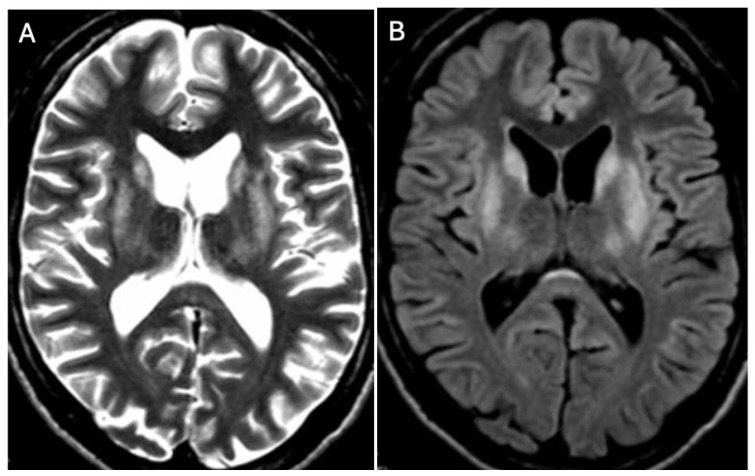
Hyperintense changes localized in putamen, head of nucleus caudate, and thalamus in T2-weighted sequences (A) and FLAIR (B) (own materials of the neurology department).
